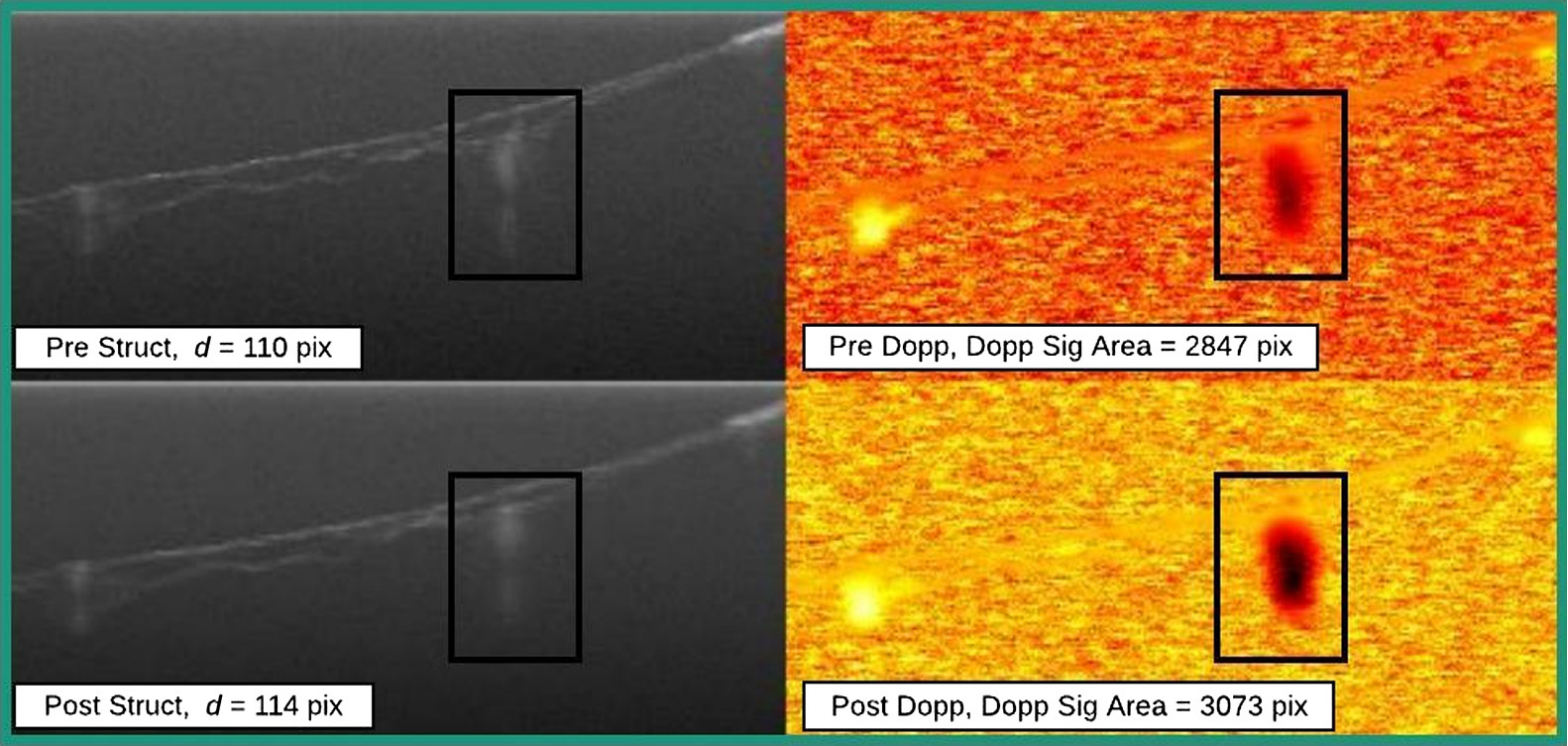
An unaffected data point. Black boxes indicate the ROIs. This data point exhibits <10% change in vessel diameter and <15% change in Doppler signal area and thus is classified as unaffected. Vessel diameters, d, are labeled in each structural image and are reported in pixels. Doppler signal area (Dopp Sig Area) is labeled in each Doppler image and is also given in pixels.
